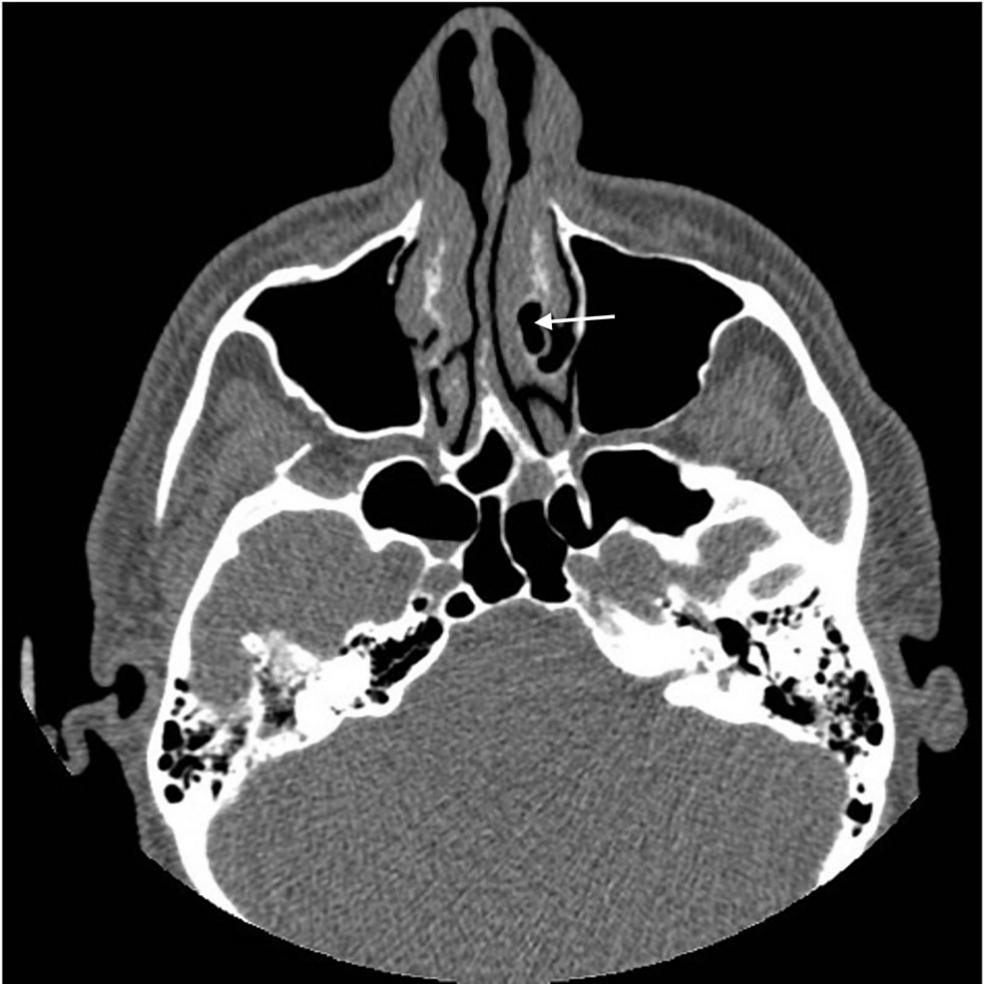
CT image of patient 2 (axial view) Axial view demonstrates CB of the left inferior turbinate (white arrow) CB: Concha Bullosa
